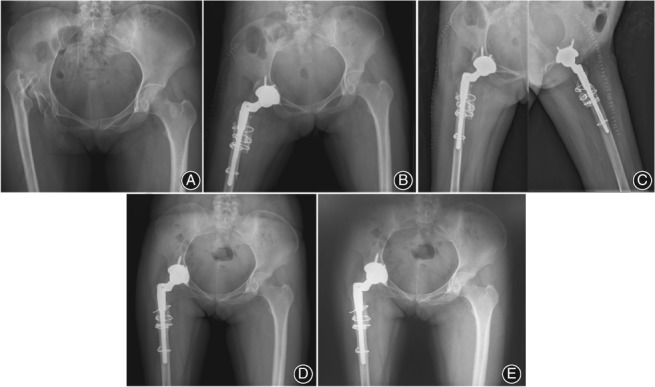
A 28‐year‐old woman with Crowe type IV high hip dislocation secondary to pyogenic arthritis who had a quiescent period of 25 years between infection and right total hip arthroscopy (THA). (A) Preoperative anteroposterior (AP) pelvic radiograph. (B) Postoperative AP pelvic radiograph immediately after right THA. (C) Postoperative AP and oblique hip radiographs immediately after right THA. (D) Postoperative AP pelvic radiograph 5 years after right THA. (E) Postoperative AP and oblique hip radiographs 8 years after right THA.
